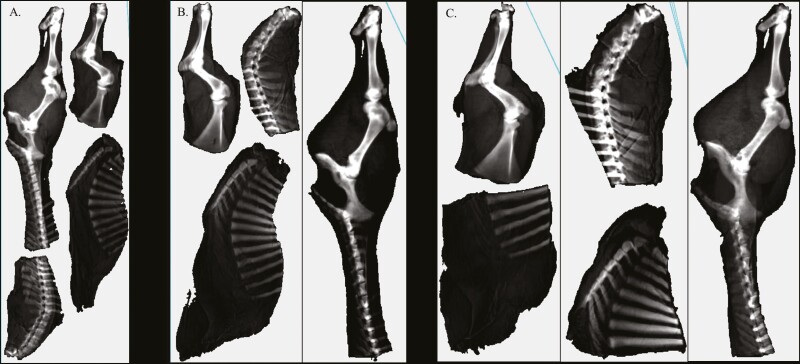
Left half-carcass cut for dual-energy X-ray absorptiometry (DXA) scans: (A) 17 to 46 kg, (B) 52 to 93 kg, and (C) heavier half-carcasses.
